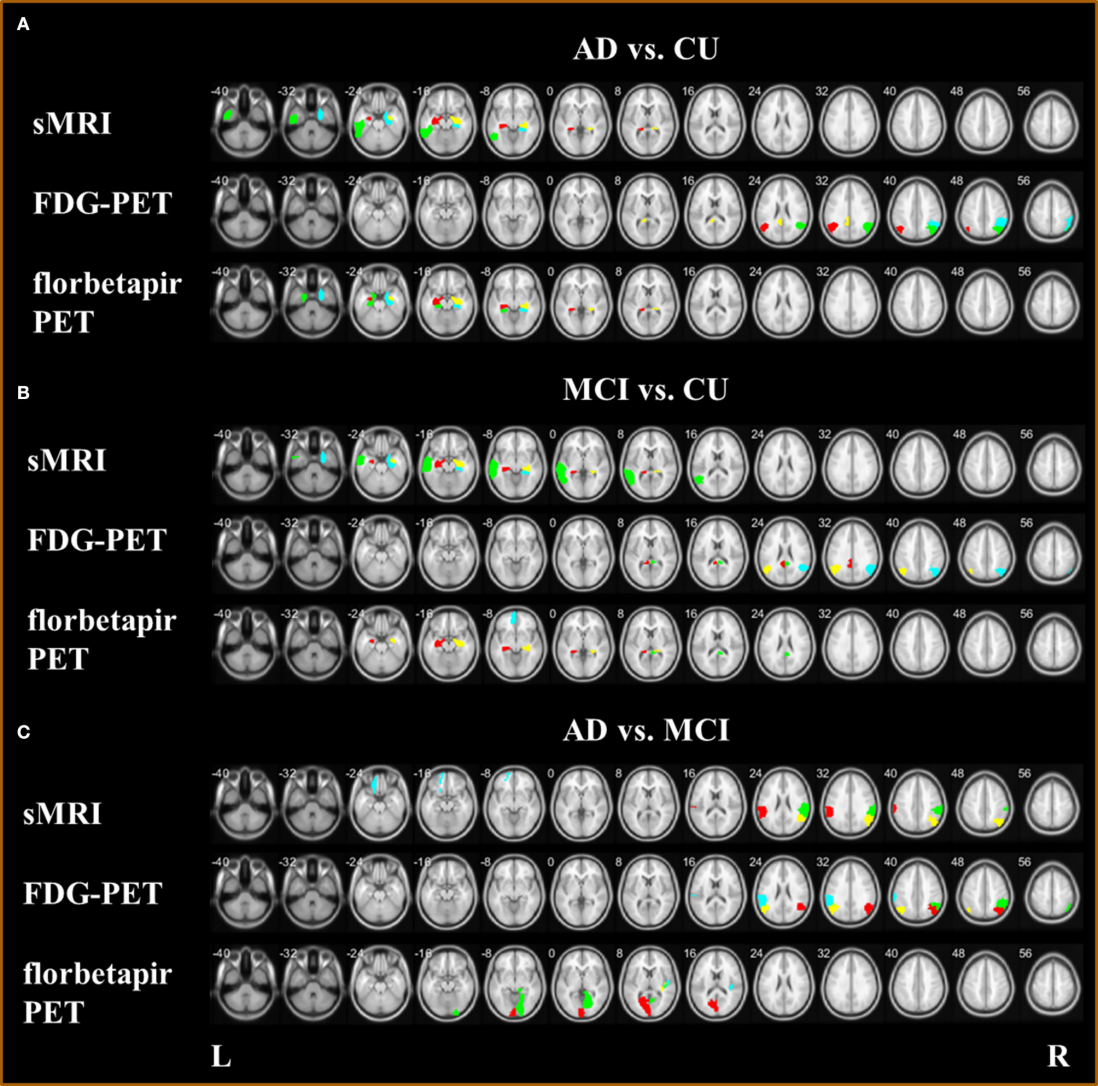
Biomarkers with sMRI, FDG-PET, and florbetapir-PET (A) for classification AD and CU; (B) for classification MCI and CU; and (C) for classification AD and MCI.
